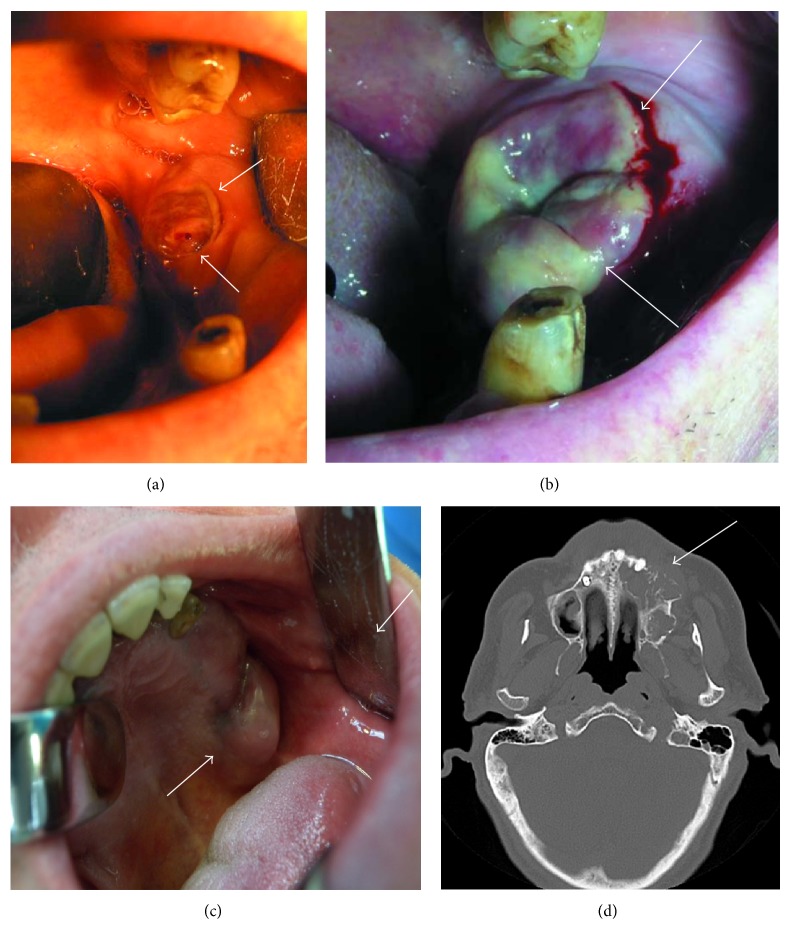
Extramedullary plasmacytoma, macroscopic features. (a) The soft mass neoplasm appears pink in colour and has floating consistency with a diameter of about 1.5 cm, occupying the area of dental elements 37 and 38. It has ill-defined contours and is slightly ulcerated on the top for the effects of antagonist dental elements, causing algia and difficulty in chewing for the patient. No sign of bone involvement at the orthopantomography (Case A). (b) The patient presented a relapse of the neoplasm seven days after the biopsy, with the enlargement of the lesion to the entire edentulous left side of the jaw, until the first premolar. The mucosa covering the lesion was of irregular colour and the traumatism due to the antagonist dental elements was accentuated (Case A). (c) The mass presented as a swelling in the left posterior region of the maxilla, with maximum dimensions 25 × 45 mm, involving also palate and gingiva. The mass consistency was hard elastic and normal in colour and covered with intact oral mucosa (Case B). (d) The maxillofacial CT scan showed an important osteolytic involvement of the upper alveolar process and the partial obliteration of the basal portion of the left maxillary sinus. The lesion extended also to the homolateral nasal cavity. Concomitant enlargement of lateral cervical and submandibular lymph nodes, with the biggest having 13 mm diameter (Case B).
